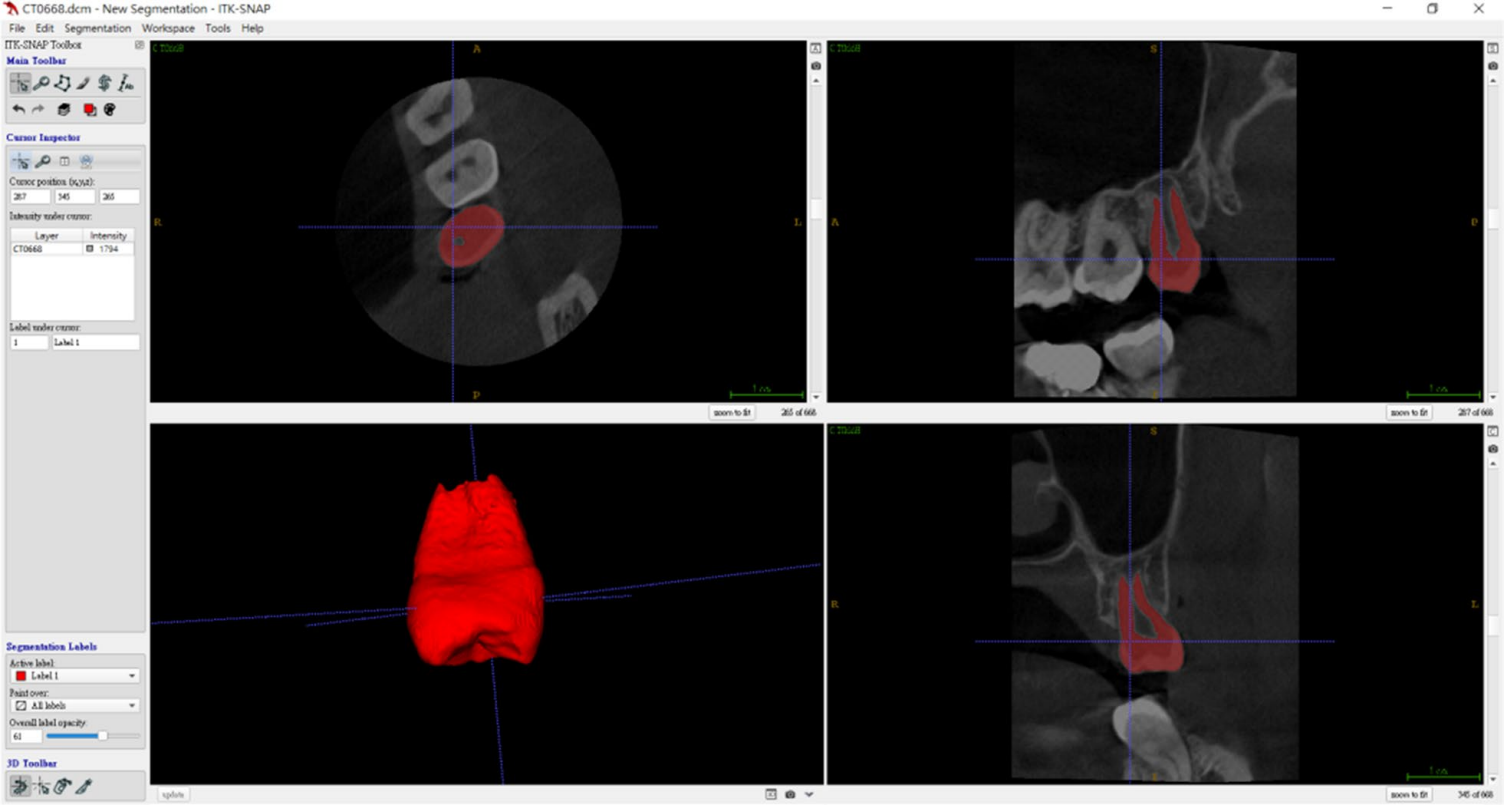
Constructing the surface mesh from the donor tooth Cone-Beam CT with the ITK-SNAP software
